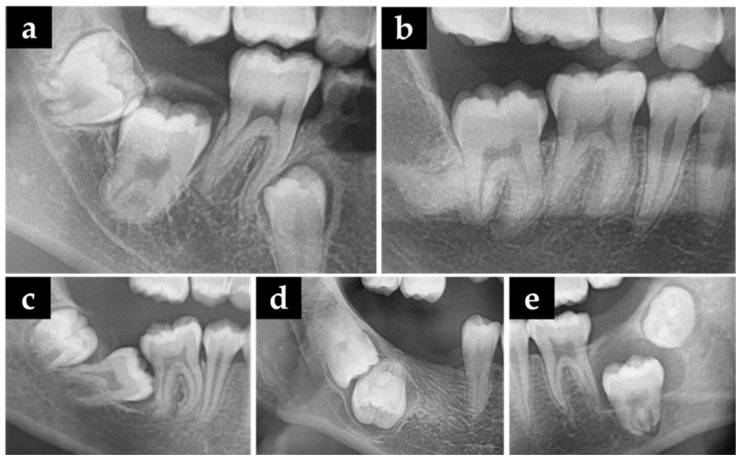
Panoramic radiographs revealed risk factors for eruption disturbances. (a) Primary retention; (b) Secondary retention; (c) Impaction due to insufficient posterior space; (d) Impaction due to ectopic eruption path; (e) Impaction due to cyst of jaw.
